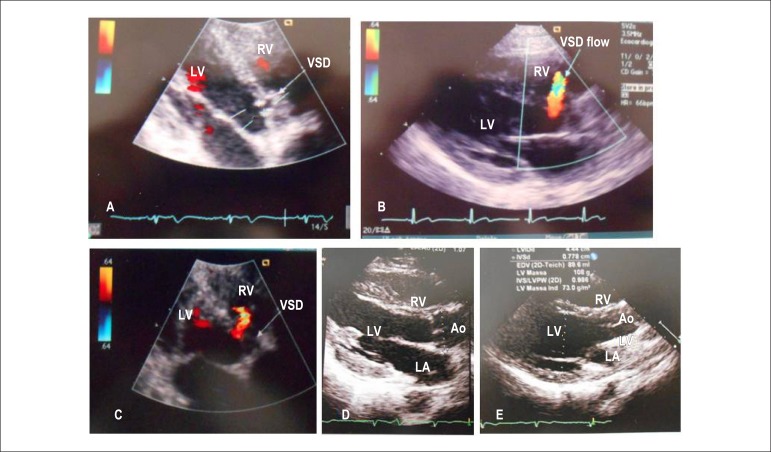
Echocardiograms showing a small subaortic ventricular septal defect at
